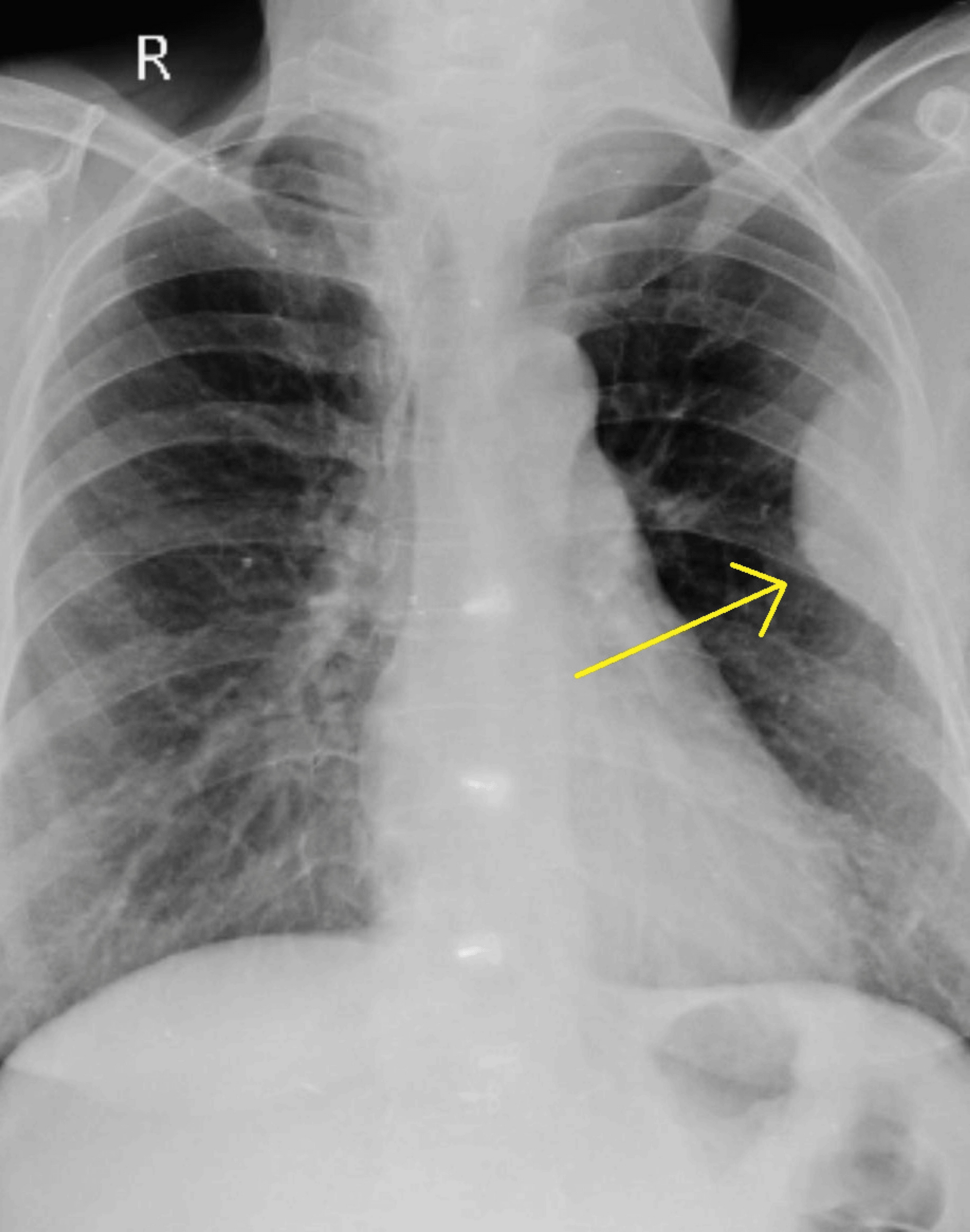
Posteroanterior view X-ray with a possibility of a pleural-based mass
